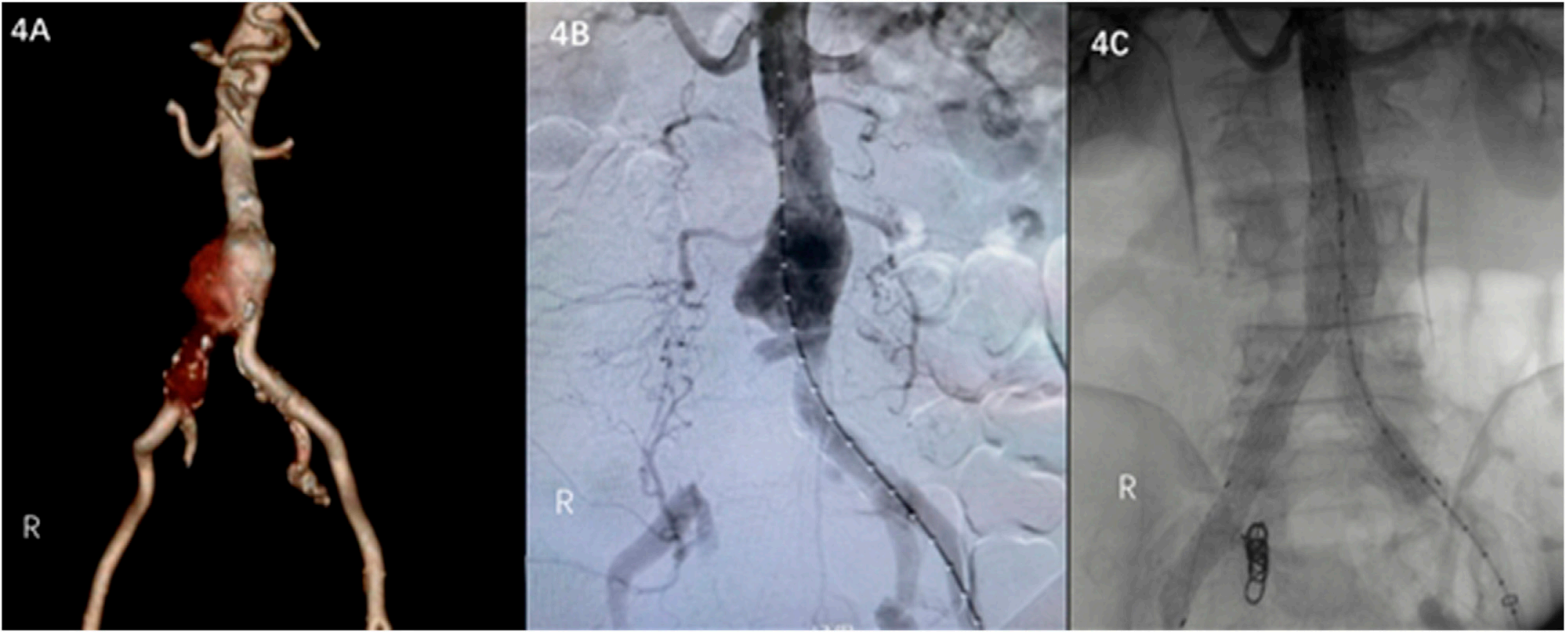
(A) Contrast-enhanced CT scan of the abdomen shows a pseudoaneurysm at the end of the abdominal aorta. (B) Intraoperative DSA shows a pseudoaneurysm at the end of the abdominal aorta and occlusion of right common iliac artery. (C) Intraoperative DSA shows no endoleaks.
